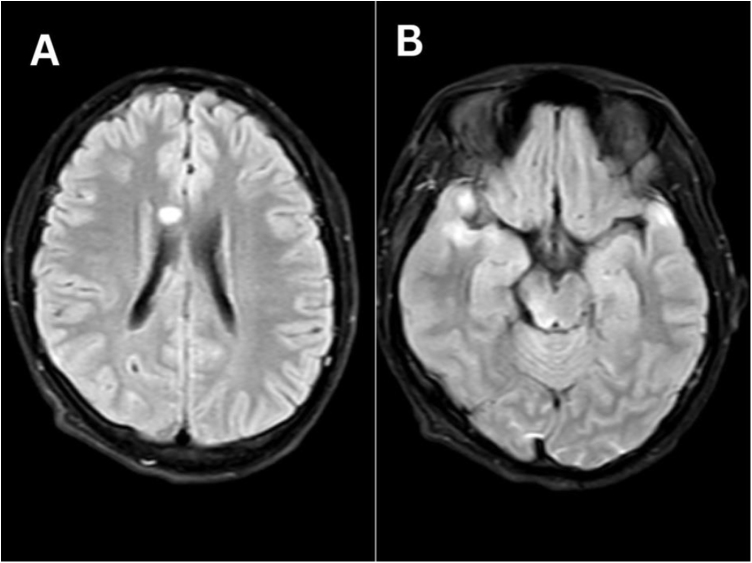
MRI brain, FLAIR, (A) showing hyperintense lesion in the corpus callosum, (B) showing hyperintense lesions in the dorsal aspect of the midbrain and cerebral cortex.
